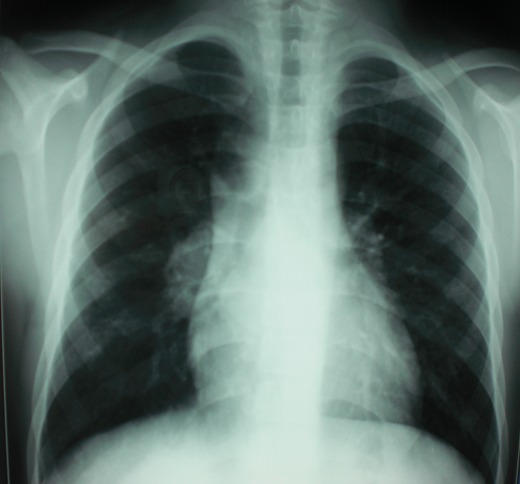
Chest radiography: superior mediastinal enlargement
